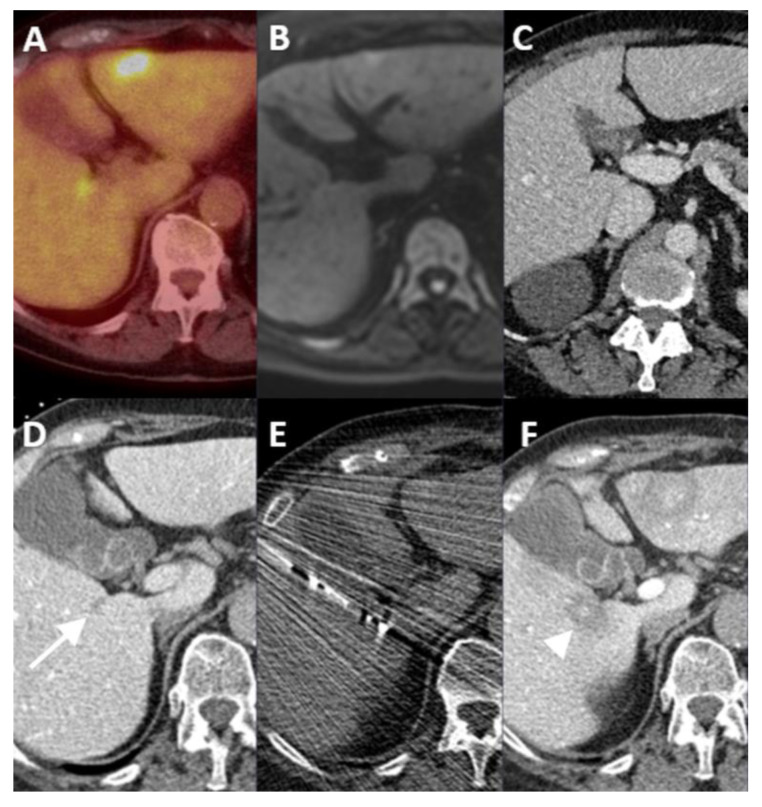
Visualization of a ‘vanished’ lesion after downstaging chemotherapy. Diagnostic ^18^F-FDG PET-CT showed two colorectal liver metastases ((A), segment III and V). Chemoradiation was given to pretreat the rectum tumor. The tumor in segment V ‘vanished’ on post-chemoradiation MRI ((B), diffusion-weight imaging, b-800) and intravenous contrast-enhanced CT (C). The patient qualified for local ablative treatment as the tumor in segment III was still visible. After catheterization of the common hepatic artery (to visualize both liver lobes) followed by the administration of 20 mL intra-arterial Xenetix 300^®^ (1:1 mixed bolus of saline and contrast agent; Iobitridol 300 mg Iodine/mL, Guerbet, Villepinte, France), the lesion (arrow) became clearly visible as a hypodense lesion of 9 mm in the portal venous phase (scanned at 22 s) (D). Percutaneous microwave ablation (Emprint™ Microwave Ablation System, Medtronic-Covidien, Boulder, CO, USA) was successfully performed (E), and the tumor was circumferentially covered by the ablation zone (arrow head) (F).
